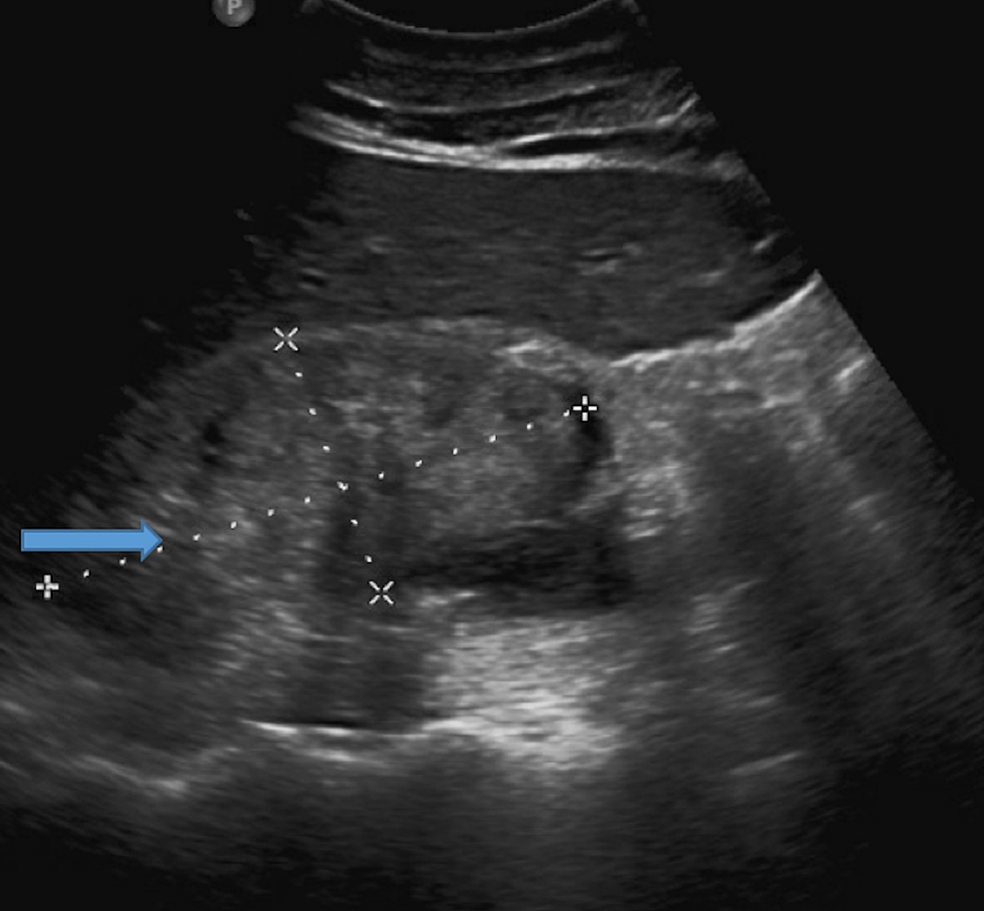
Ultrasound of the right kidney (blue arrow) measuring 9.61 by 4.61 cm.
